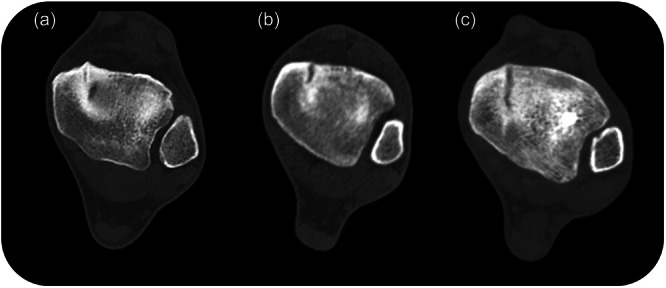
Axial view of computed tomography scans of the ankle with a medial malleolus stress fracture in various stages. (a) Stress fracture confined to the anteromedial osteophyte; (b) stress fracture progressing beyond the anteromedial osteophyte; (c) stress fracture encompassing 50% of the medial malleolus in the sagittal plane.
